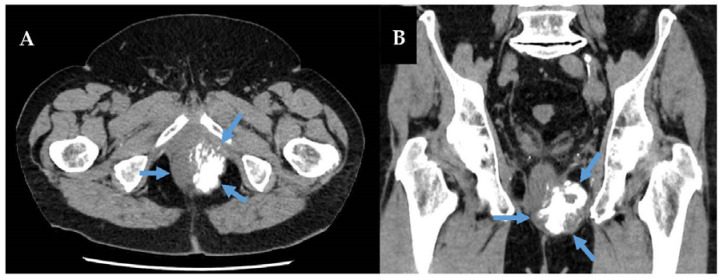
Primary retroperitoneal extraosseous osteosarcoma (blue arrows) axial (Panel (A)) and coronal (Panel (B)) views. This tumour shows CT characteristics including a deep-seated pelvic tumour with significant calcification matrix, and invasion into the rectum, prostate, pelvic floor muscles, and left pelvic sidewall. This patient underwent R0 resection of RPS with pelvic exenteration including en bloc extralevator abdominoperineal resection, cystoprostatectomy, clearance of ischiorectal fossa and ischial tuberosity without bony involvement.
